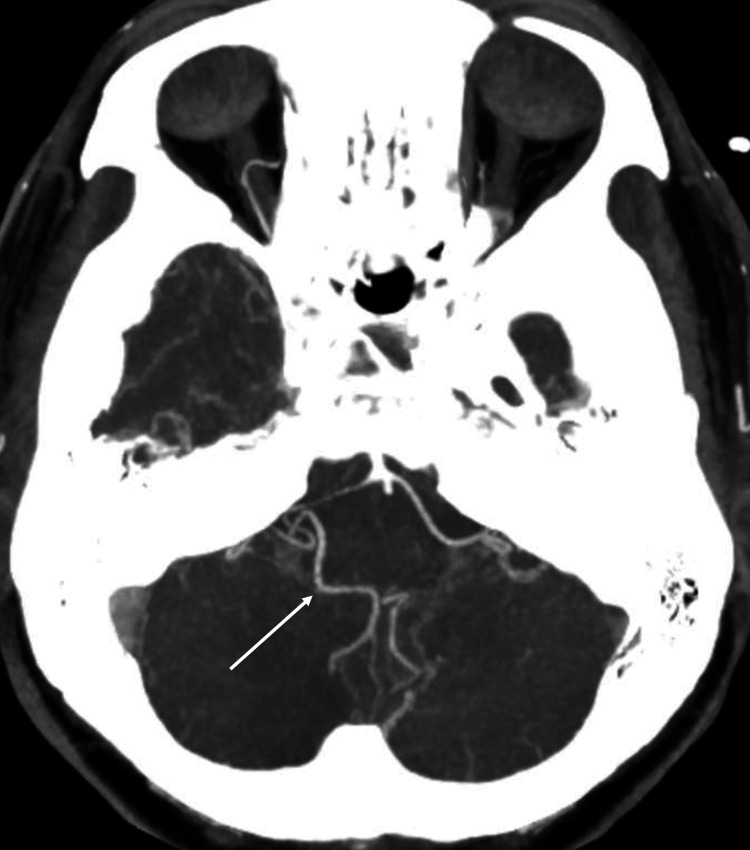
Post-procedure computed tomography angiography demonstrating continued preservation of the posterior inferior cerebellar artery (white arrow)
